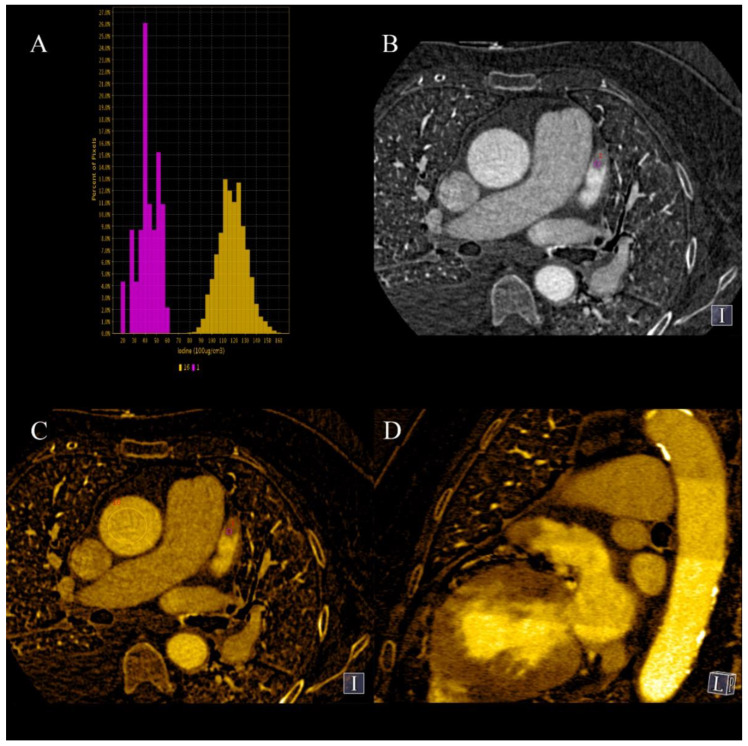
(A–D). Case of patient with chronic atrial fibrillation after 3 unsuccessful ablations, currently admitted due to chest pain. Coronary CTA was performed to roll out coronary artery stenosis. Differentiating thrombus and filling defect of LAA using iodine concentration is much more specific and sensitive than the use of Hounsfield unities ratio, as proven by Hur et al. [64]. (A)—Histogram of iodine concentration in ROIs; (B)—map iodine(water) shows lower concentration of iodine in LAA of about 4.1 mg/mL, meeting the criteria for circulatory stasis by Hur et al.; (C,D)–70 keV VMI with color overlay of iodine(water) map. (D)–in log axis of LAA. In the lower right corner of sub-image B-C-D information about viewpoint.
